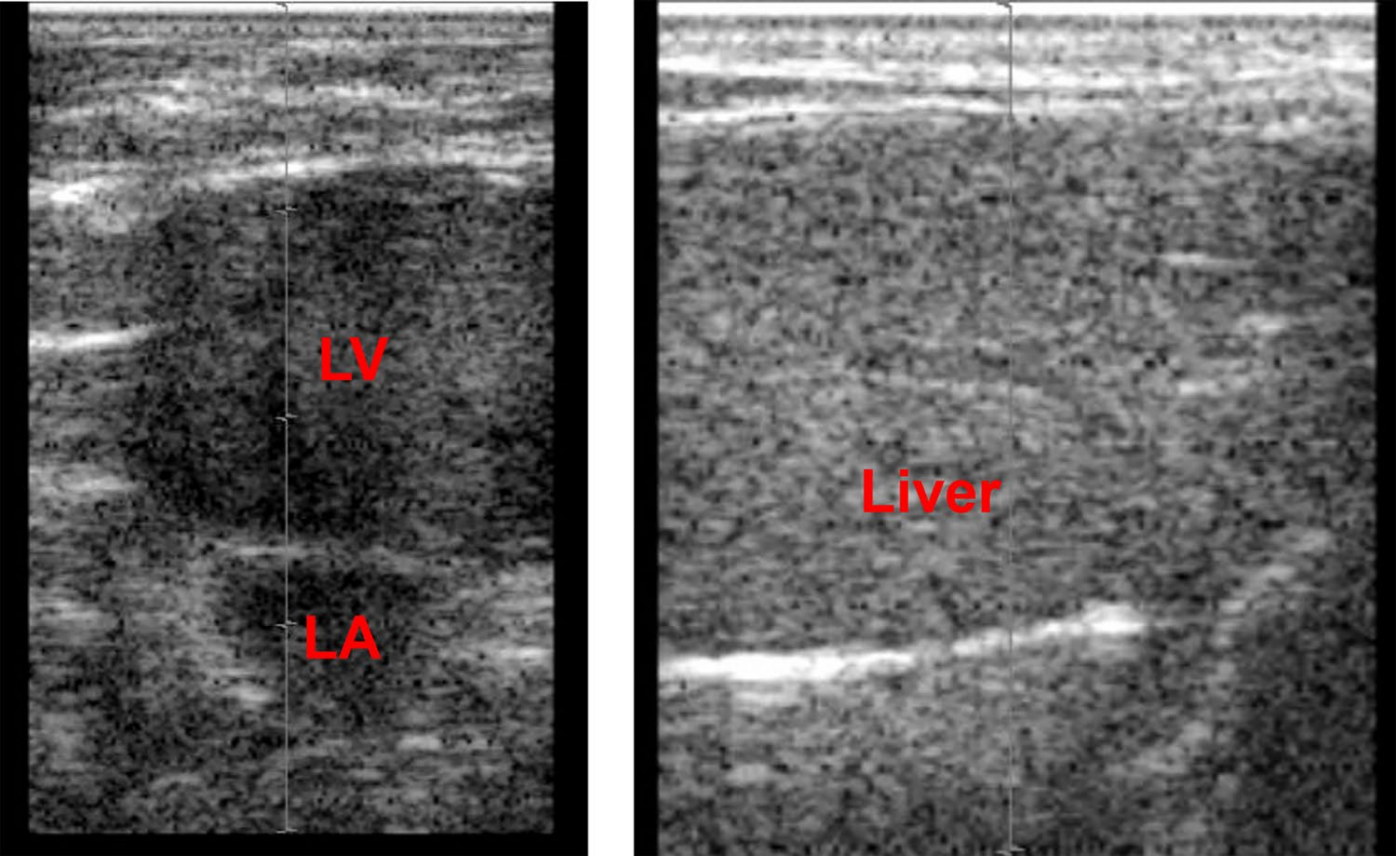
The presence of UCA in the tissue was confirmed under B-mode observation of the heart and the liver. *UCA* ultrasound contrast agent. Left: Sonazoid was detected as high-echoic blood flow in the cardiac chambers. *LV* left ventricle, *LA* left atrium. Right: Sonazoid was detected as high-echoic liver parenchyma where Sonazoid was taken up by Kupffer cells
